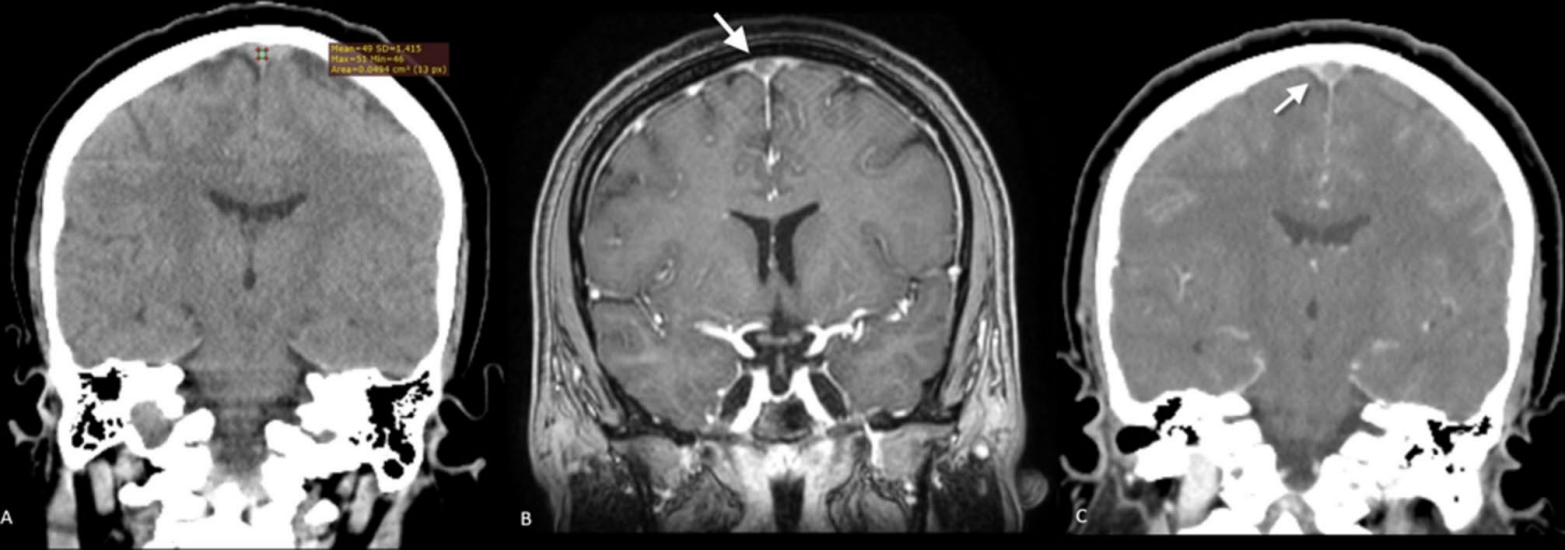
A–C Superior sagittal sinus (SSS) of a 36 year-old male appeared normal on non-contrast computed tomography with an average attenuation of 49 HU (A) and was interpreted as normal. However, contrast-enhanced CT in coronal (B) and CT venography in sagittal (C) reconstruction showed a well-defined filling defect within the SSS (arrows) consistent with thrombosis of the SSS. As the patient’s hematocrit was within normal range and the patient presented only by headache, a possible explanation for the false negative measurements may be a significant time interval between the onset of symptoms and imaging, by which time the thrombus elicited lower attenuation values
